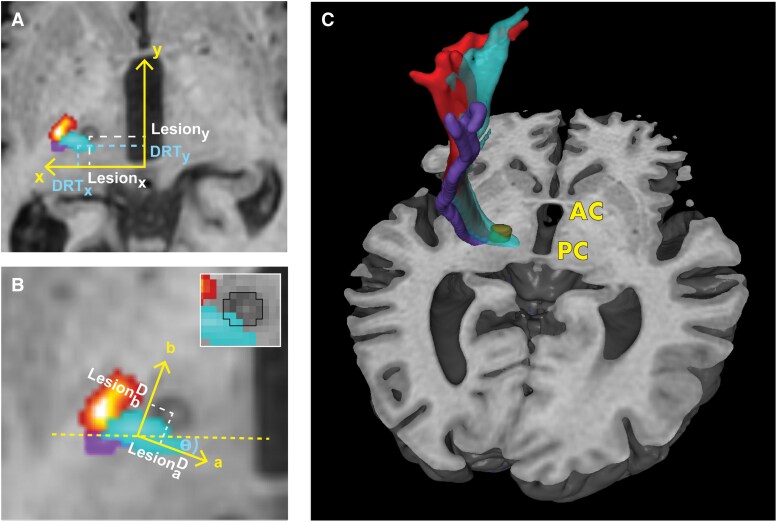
Lesion evaluation with respect to the DRT (cyan), PT (red), and ML (purple) using two coordinate systems. (A) AC-PC coordinate system, origin at the PC point. Positive × toward the treated thalamus. (B) DRT coordinate system normalized to the ellipse long axis (rotated by Ѳ). Origin at the DRT COM. Positive a toward the midline. Note, lesion geometry analysis at T1 MRI (B). (C) 3D visualization of the three tracts and the lesion (dark yellow). Lesion^D^, Lesion’s COM coordinates with respect to the DRT coordinate system. AC, anterior commissure; DRT, dentatorubrothalamic tract; ML, medial lemniscus; PC, posterior commissure; PT, pyramidal tract.
